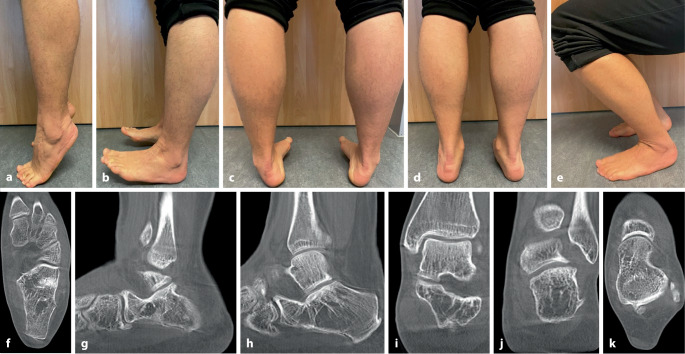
18-Jahres-Follow up: *Klinisch *sind Zehenspitzen- und Fersenstand (a,b), Inversion und Eversion (c,d) seitengleich aktiv ausführbar. Bei maximaler Kniebeuge (e) ist kein Einkrallen der Zehen links feststellbar. *Radiologisch *zeigt das CT im Stehen: Das linke Fersenbein hat eine normale, achsengerechte Form (f). Das Kalkaneokuboidalgelenk (g,h) hat normale Weite und ist nahezu völlig kongruent. Das Kuboid ist weiter medial (h) gering verkürzt fehlverheilt und plantar nach posterior ausgezogen. Die mediale Facette (i) ist normal weit, das Sustentaculum tali anatomisch verheilt. Die posteriore Facette (j) ist nahezu anatomisch verheilt, die subtalare Gelenkweite (h,j) normal. Der Talus (k) ist in Achse und Form normal verheilt, ebenso das nicht gezeigte Metaltarsale V
